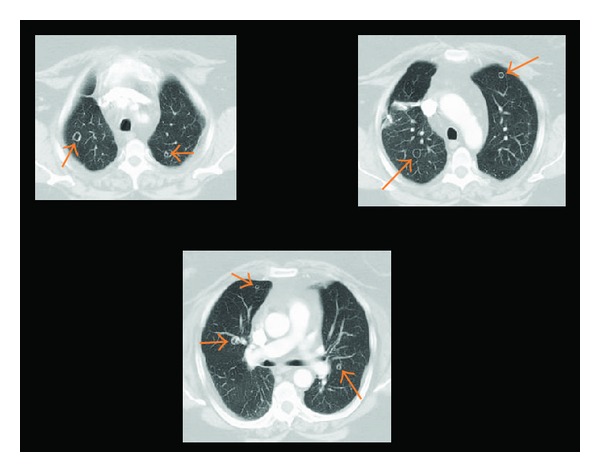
CT: multiple bilateral cavitary pulmonary nodules.
